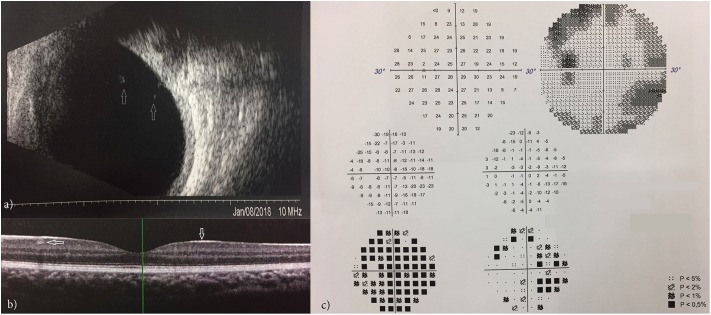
Ocular ultrasonography showing vitreous condensation (white arrows) (a); OCT showing a hyperreflective inner limiting membrane with hyperreflective foci within the ganglion cell layer (b); VFT showing points of reduced retinal sensitivity (c).
